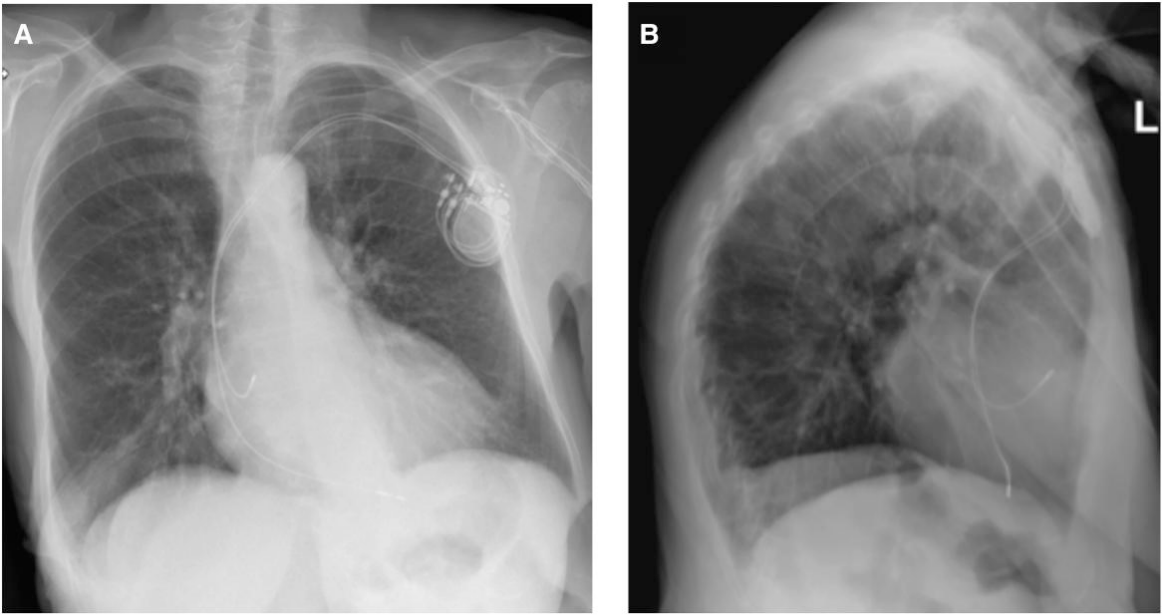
A chest X-ray following PM implantation revealed no evident abnormalities on either posteroanterior view (*A*) or lateral view (*B*).
